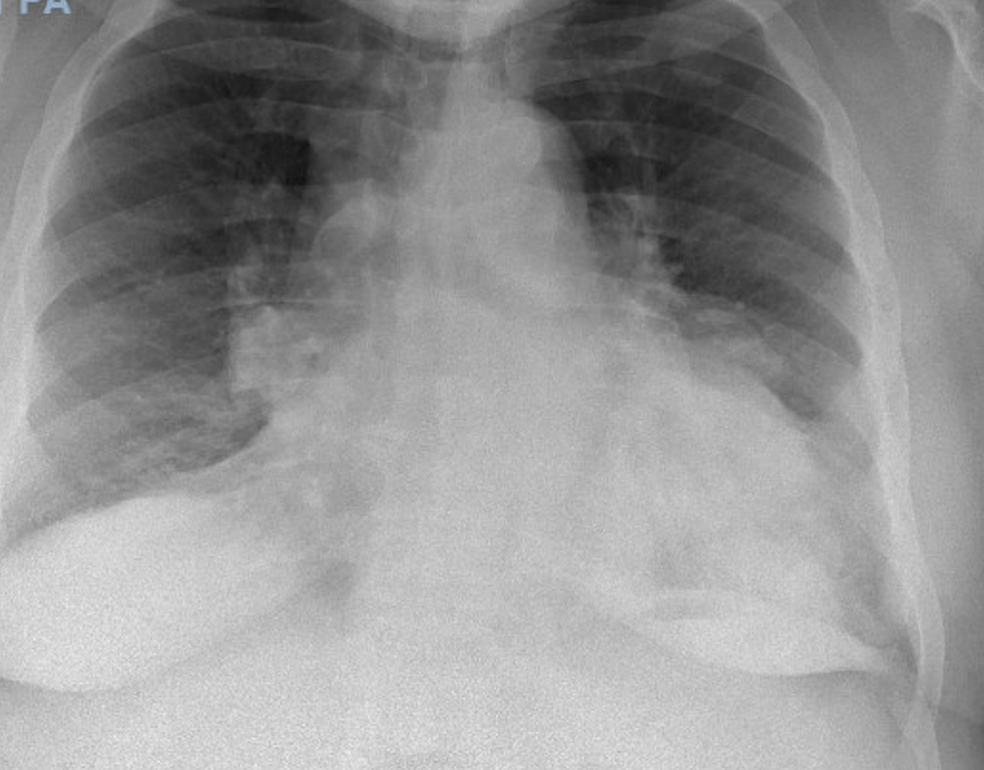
Chest X-ray. Patchy opacities in the left lung base and mild prominence of the bilateral hilar nodes.
